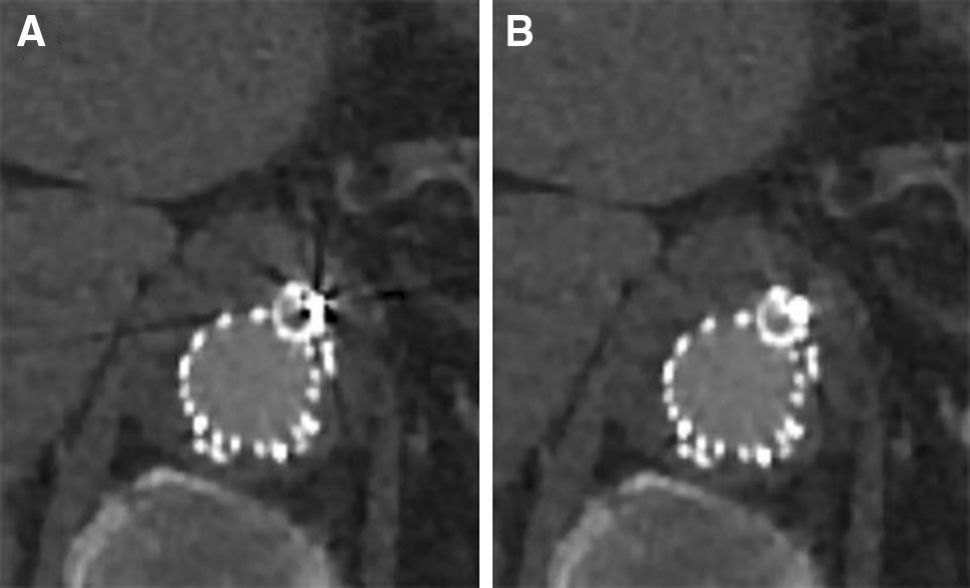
CT images (A standard (AIDR 3DE), B SEMAR) of a branch connected to the celiac artery in a 65-year-old male. Artifacts (black streaks) are reduced on the CT image with the SEMAR reconstruction compared to the standard reconstruction. All CT images are displayed with a window level of 300 HU and a window width of 1000 HU
